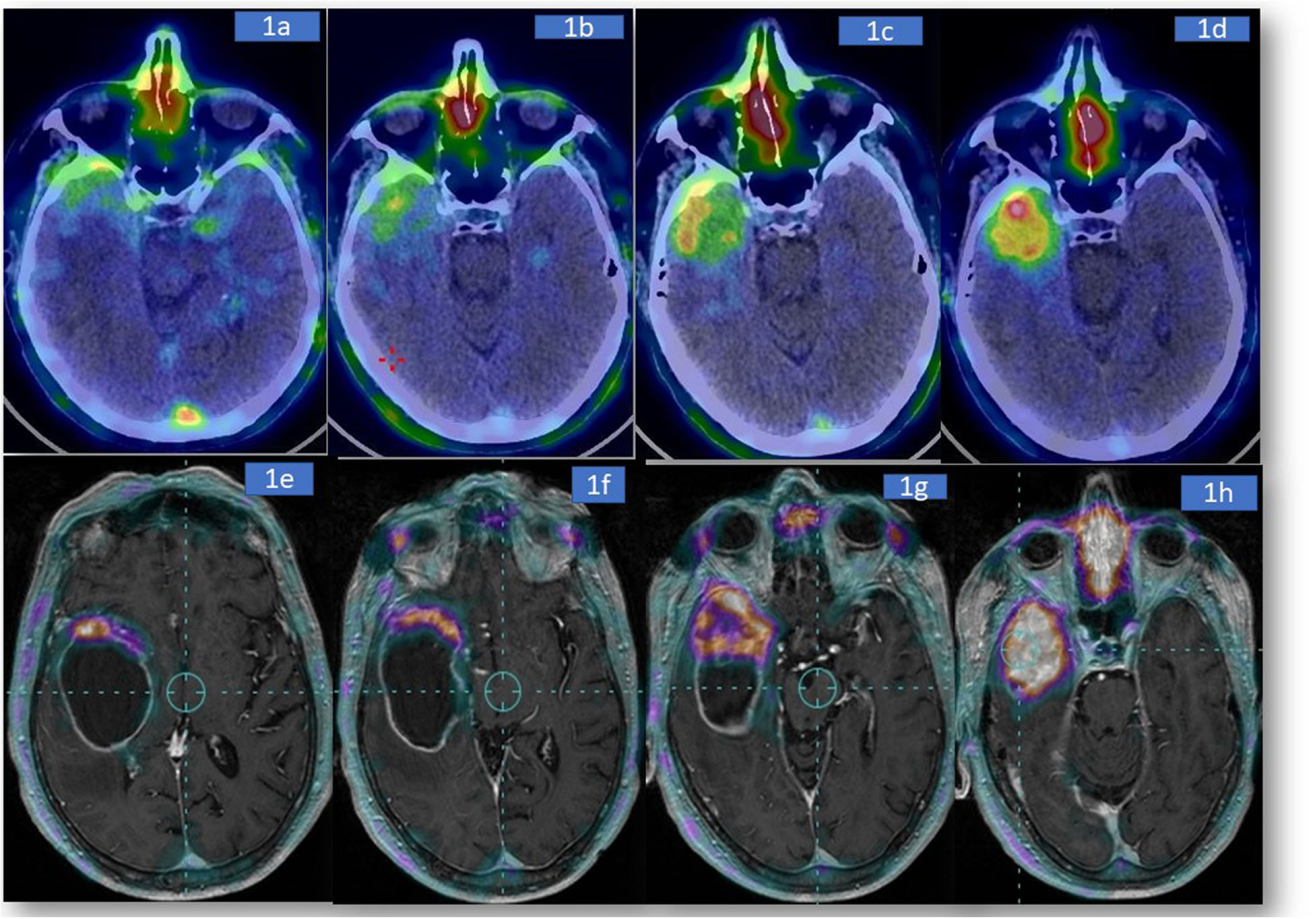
PET/CT acquisition at different times after injection. Brain images taken at 5 min (a) 1, (b), 4 (c) and 18 h (d) post-radiotracer injection. Progressive and significant uptake of [^64^Cu][Cu(ATSM)] into the lesion is documented during time. The SUVmax increases from 2.0 (5 min post-injection) to 4.9 (18 h post-injection). The heterogeneity of tumour is particular evident on PET-MRI fusion images obtained at 18 h. Images e-h represent different transaxial planes in cranio-caudal direction (upper (e) to lower (h) planes)
